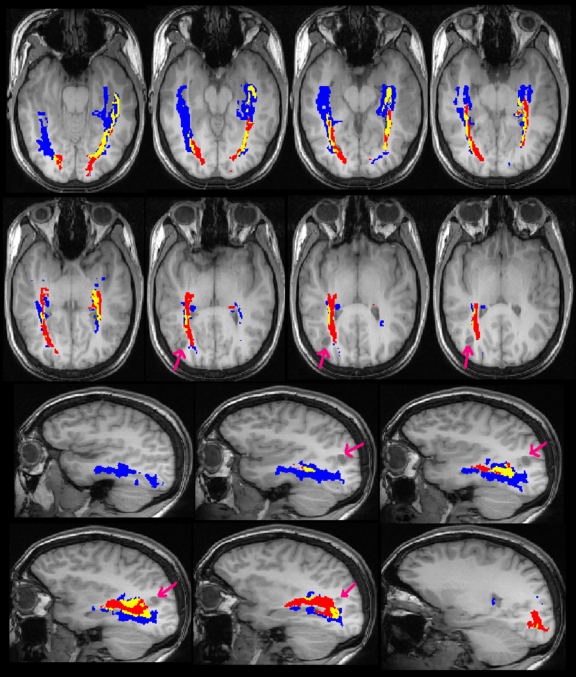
Optic radiation identified in patient 1 by Method 1 only (blue), Method 2 only (red) and both methods (yellow), overlaid on the T1-weighted image. The arrows indicate the right inferior parietal DNET. Image conventions as Fig. 3.
